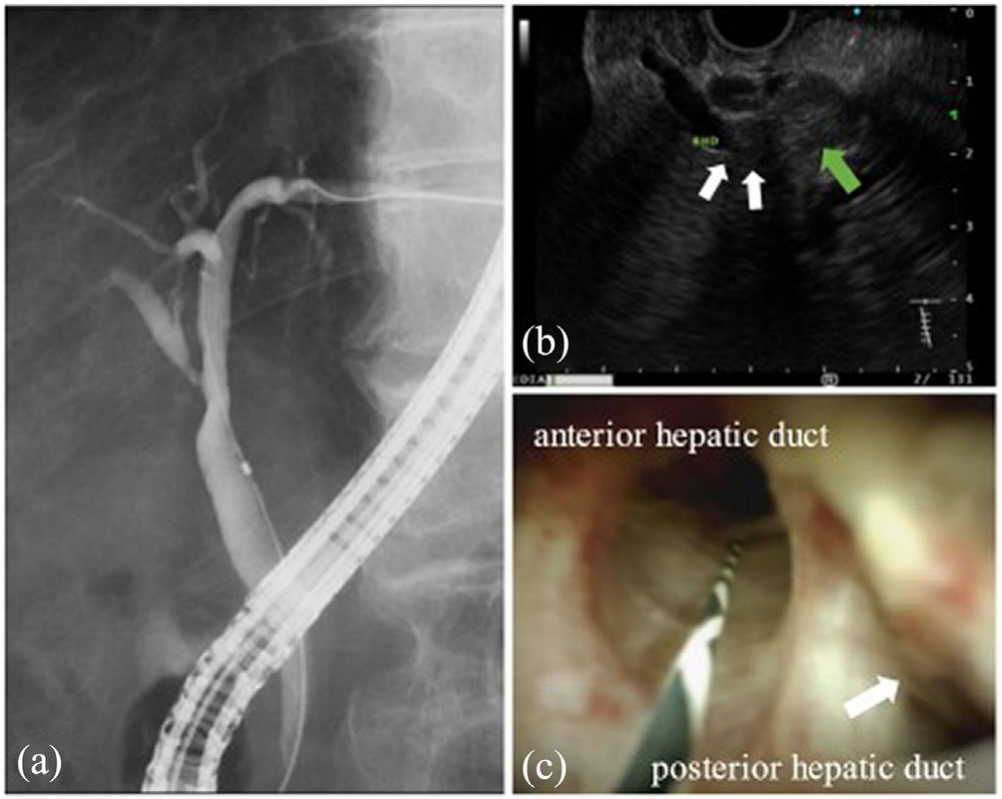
(a) Endoscopic retrograde cholangiopancreatography: stenosis of the right anterior segment bile duct and failure to visualize the posterior segment bile duct. (b) Endoscopic ultrasound: The bile duct was filled with substantial structures (white arrow) and was contiguous with the mass in the posterior segment (green arrow). (c) Peroral cholangioscopy: A villous mass was observed inside the posterior hepatic duct (white arrow); it was difficult to observe from the front. No tumor was observed at the anteroposterior bifurcation of the right hepatic duct to the left‐right hepatic duct bifurcation.
